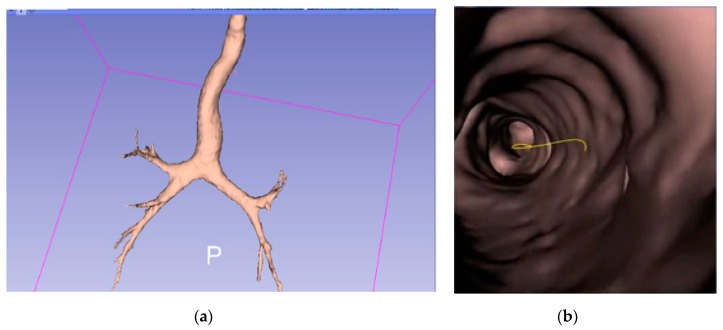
(a) Virtual 3D bronchial model constructed from computed tomography (CT) slices; (b) a sample virtual bronchoscopy image.
